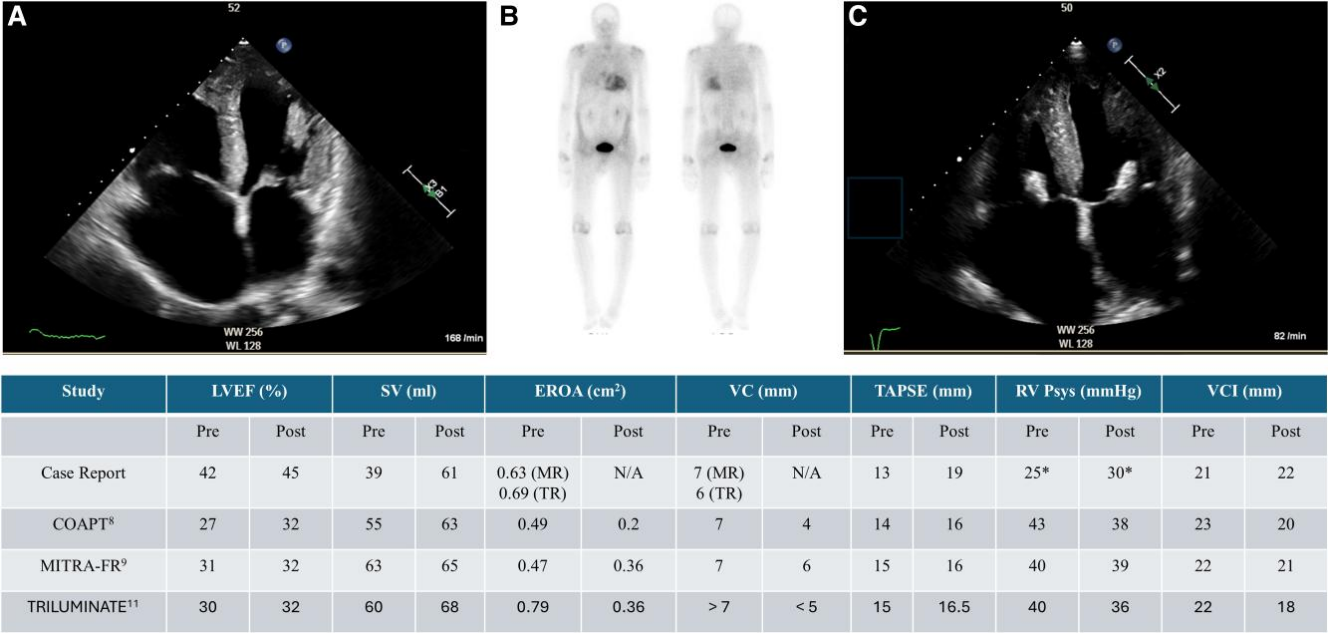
(*A*) TTE four-chamber view before MV and TV TEER. (*B*) Scintigraphy Perugini grade 2. (*C*) TTE four-chamber view after MV and TV TEER. EROA, effective regurgitant orifice area; LVEF, left ventricular ejection fraction; MV, mitral valve; MR, mitral regurgitation; SV, stroke volume; RV Psys, right ventricular systolic pressure; TEER, transcatheter edge-to-edge repair; TTE, transthoracic echocardiography; TV, tricuspid valve; TR, tricuspid regurgitation; VCI, vena contracta inferior; VC, vena contracta. *Potential underestimation due to concomitant severe TR. Data shown from Goel *et al*.,^8^ Obadia *et al*.,^9^ and Sorajja *et al*.^10^
